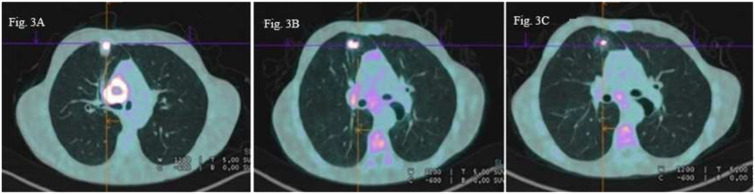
18F-FDG PET scan images axial sections (A) Right upper lobe pulmonary primary lesion (26×19 mm) with right hilar necrotic adenophaty (B) Partial metabolic regression of the primary lung lesion (10 mm versus 26 mm and the right hilar lymph node (C) Persistent partial metabolic response of the pulmonary lesion and hilar lymph node.
